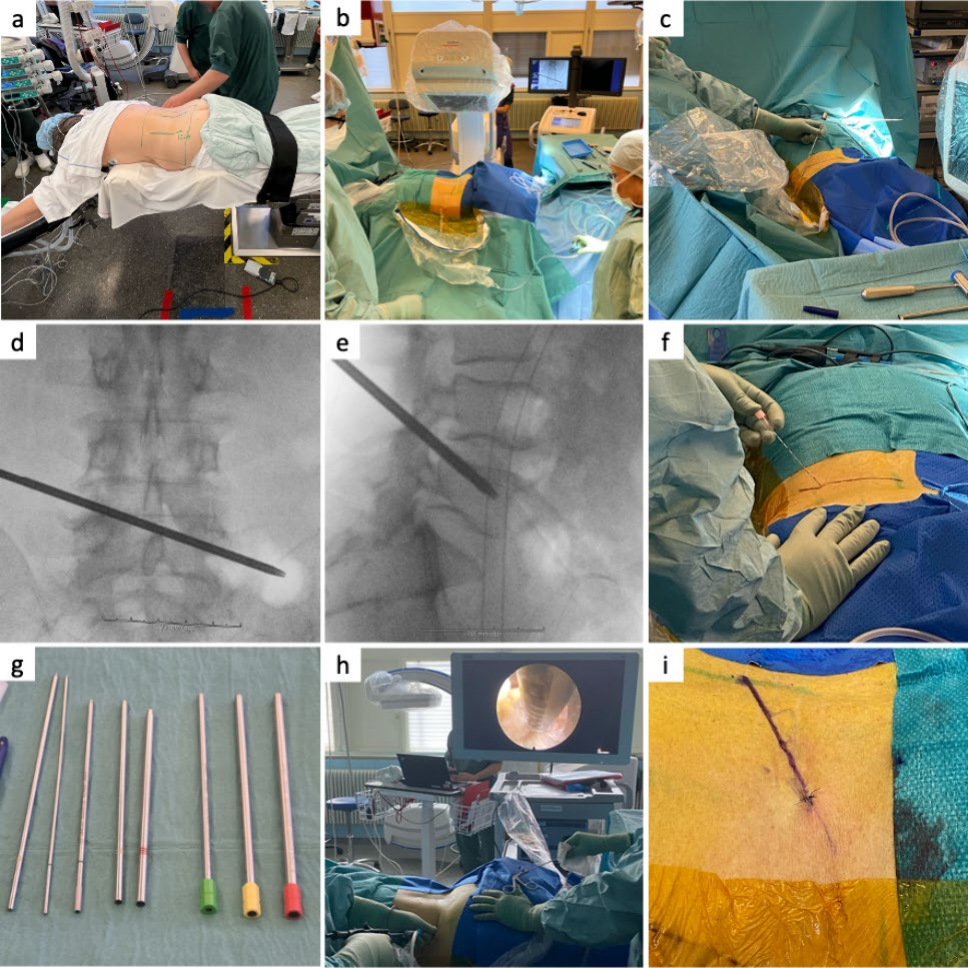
Images of a lumbar percutaneous endoscopic discectomy showing a patient in prone position (a); the surgeon, nurse, and instruments setup (b); the marking of the anterior–posterior and the lateral line using a steel rod and X-ray (c); the marking of the anterior–posterior line (d); the marking of the lateral line (e); the insertion of the 18-G needle (f); the dilatators and reamers used to widen the intervertebral foramen (g); the removal of loose tissue and herniated fragments under full endoscopic view (h); and the skin closure with a single suture (i)
